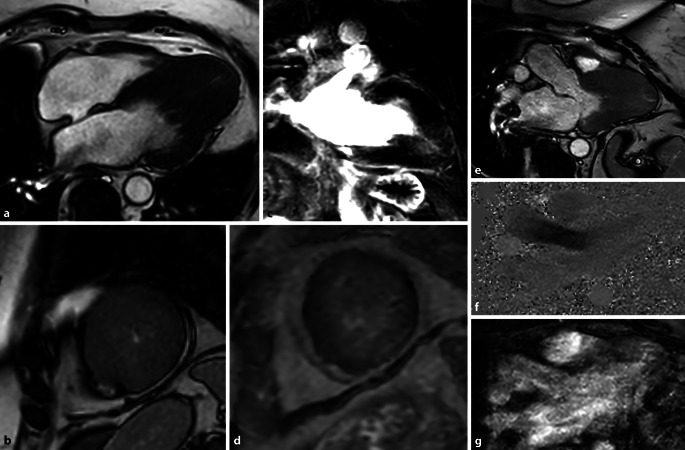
Die Obstruktion des LVOT wird zunächst visuell beurteilt und dann, meist besser objektivierbar, auch funktionell mittels der Doppler-Echokardiographie oder seltener der Phasenkontrastflussmessung in der MRT (Abb. 3f, g). In den Cine-Sequenzen sieht man durch die Flussbeschleunigung Dephasierungsartefakte im LVOT und eventuell eine systolische Vorwärtsbewegung des anterioren Mitralklappensegels, im Englischen „systolic anterior movement“ oder SAM-Phänomen genannt.
